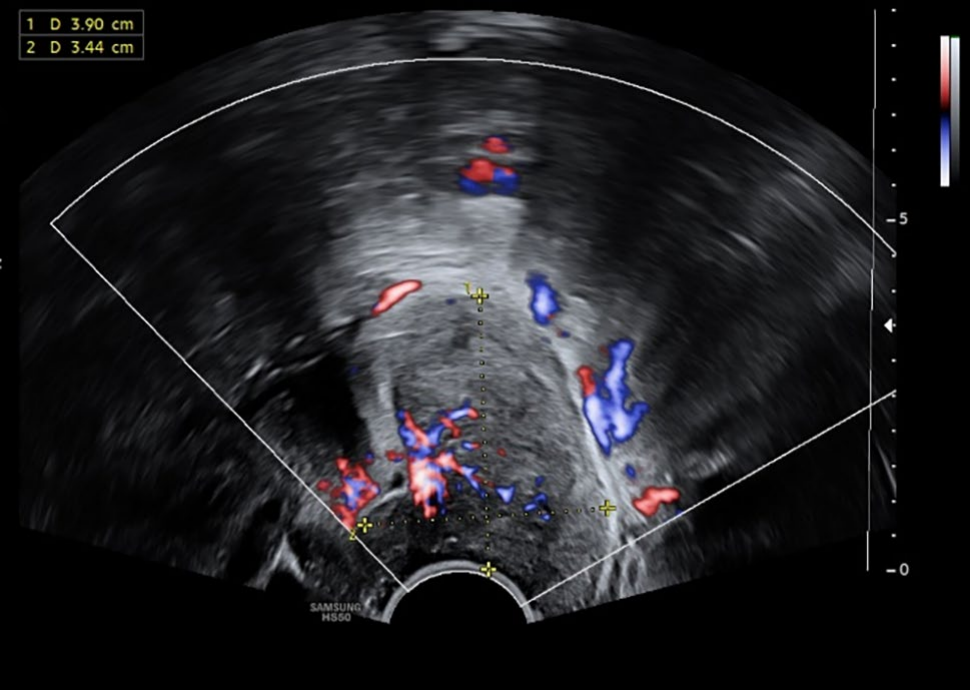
Transvaginal duplex sonography shows intensive vascularization of the tumor of the uterine cervix
